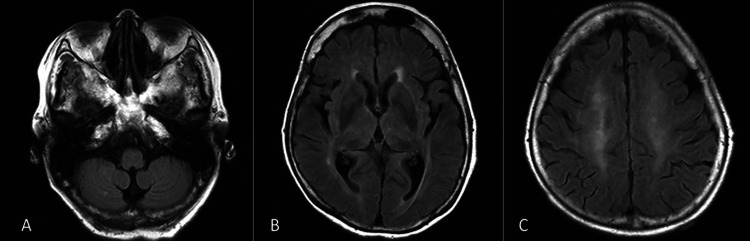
MRI of the brain. An axial fluid-attenuated inversion recovery-weighted (1.5 T; TR, 4,000 ms; TE, 106 ms) MRI of the brain shows the normal signal in (A) dentate nuclei, (B) thalamus, and (C) cerebral cortex. MRI, magnetic resonance imaging; TR, repetition time; TE, echo time
